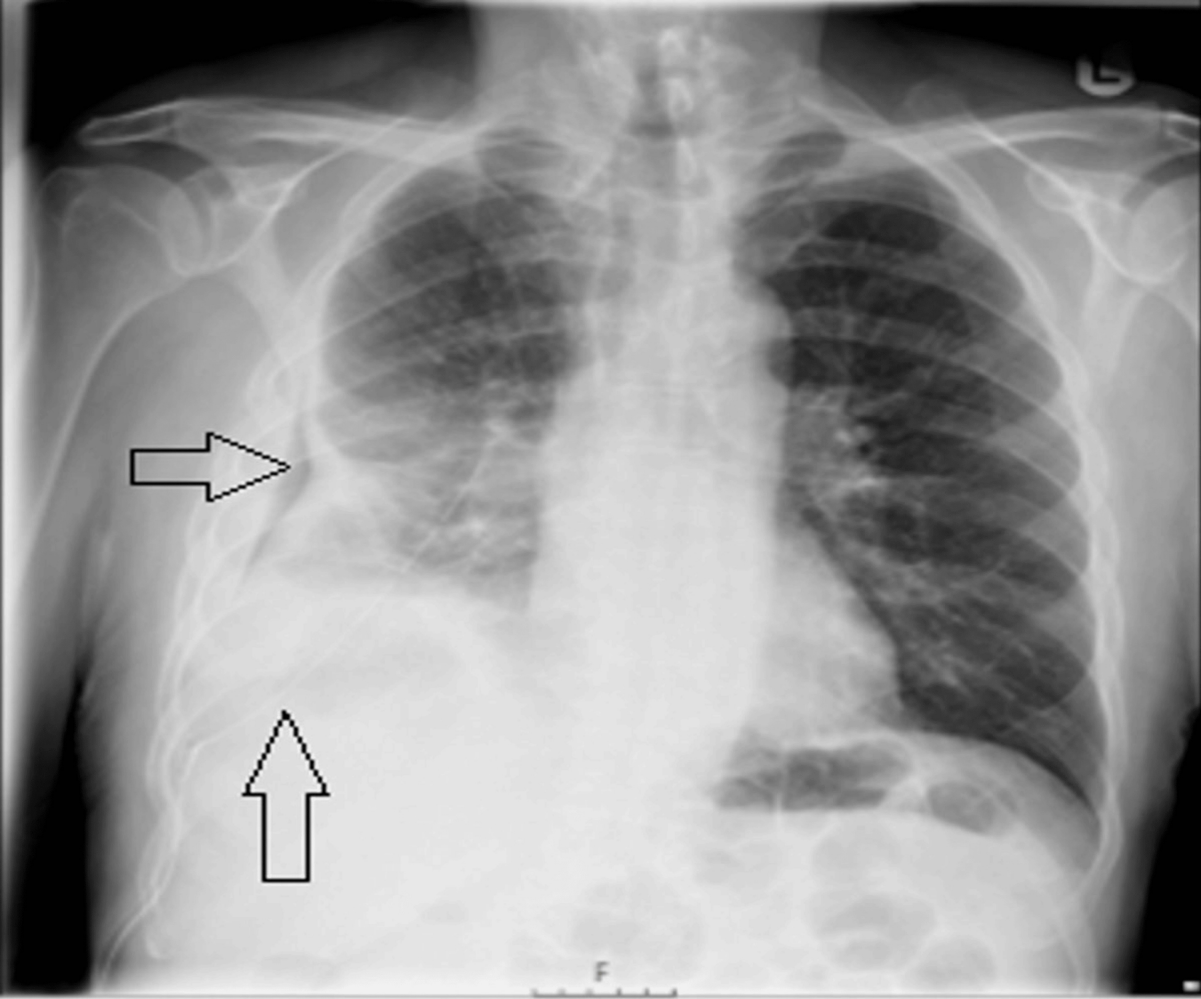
The chest X-ray showed the chest drain in situ, with significant pleural thickening and pneumothorax ex vacuo attributed to a non-expandable lung due to extensive visceral pleural rind.
